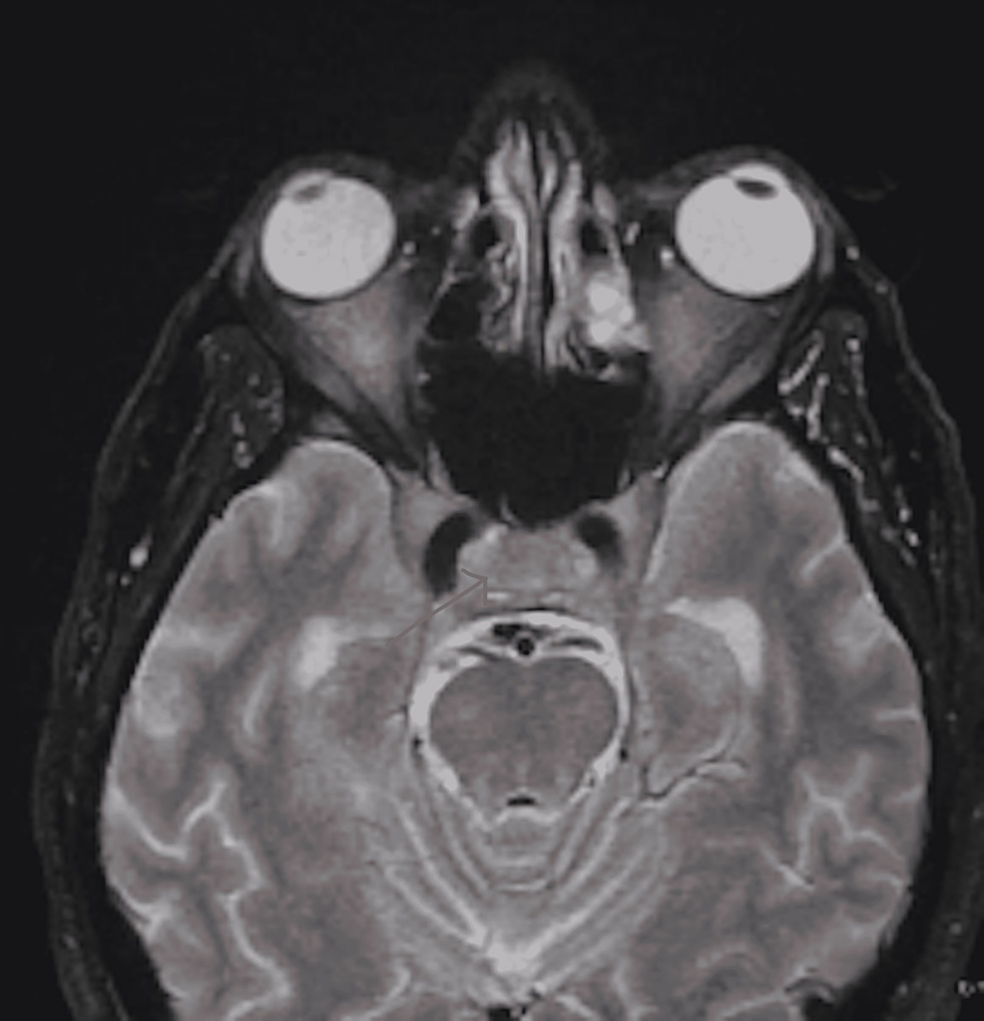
Pre-contrast T2 MRI Brain Demonstrating Diffuse Abnormal Thickening and Enhancement of the Cavernous Sinus
